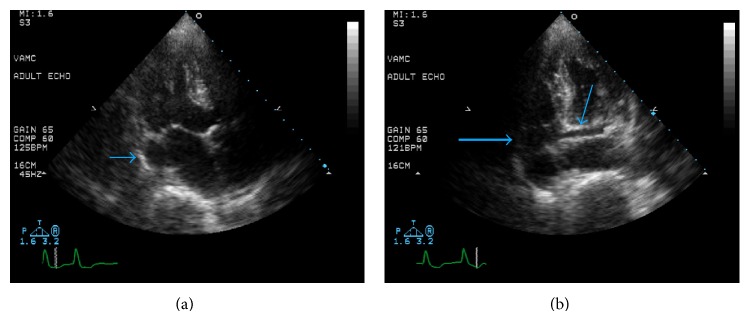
(a) Echocardiogram showing an off-axis longitudinal view of a portion of the persistent left superior vena cava (arrow) traversing under the left atrium on its course to the coronary sinus. (b) Echocardiogram showing an off-axis cross-sectional view of persistent left superior vena cava (large arrow) before it terminates in the dilated coronary sinus (small arrow).
